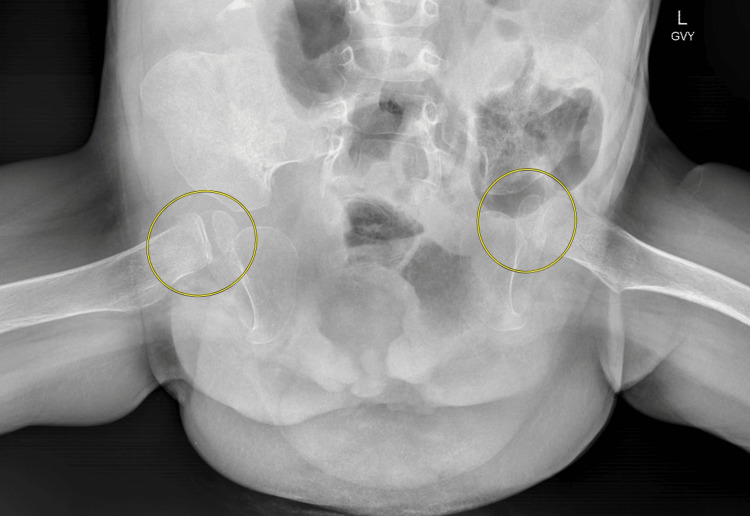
Pelvic X-ray after bilateral pelvic osteotomies (yellow circles)
